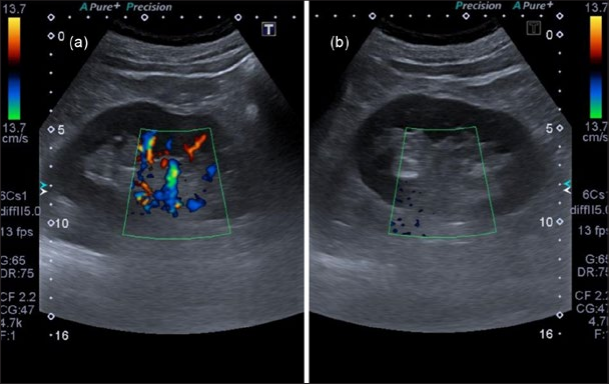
Doppler-mode ultrasound of right and left kidney. 1a: Normal vascular signal at the hilum of the right kidney. 1b: Absent vascular signal at the hilum of the left kidney with the same ultrasound parameters.
